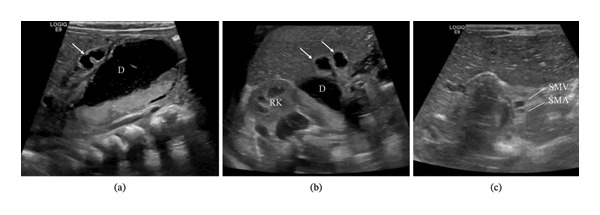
Limited abdominal ultrasound to assess for midgut volvulus. (a) Longitudinal grayscale image of the right upper quadrant near the gallbladder fossa shows a fluid‐distended viscous structure consistent with the dilated descending duodenum (D) containing layering intraluminal contents. Immediately adjacent to this was a tubular structure with anechoic contents (arrow) and wall folds, suggestive of a dilated cystic duct at the time of ultrasound interpretation. (b) Transverse grayscale image at the same level shows two parallel‐coursing tubular structures in the expected location of the gallbladder fossa (wide and narrow arrows), presumed to represent a small gallbladder and a distended cystic duct but later discovered to be a Type II choledochal cyst adjacent to the gallbladder at surgical exploration. RK = right kidney. (c) Transverse grayscale image of the upper mid abdomen slightly caudal to the above image shows an abnormal configuration of the superior mesenteric vein (SMV) immediately anterior to the superior mesenteric artery (SMA).
